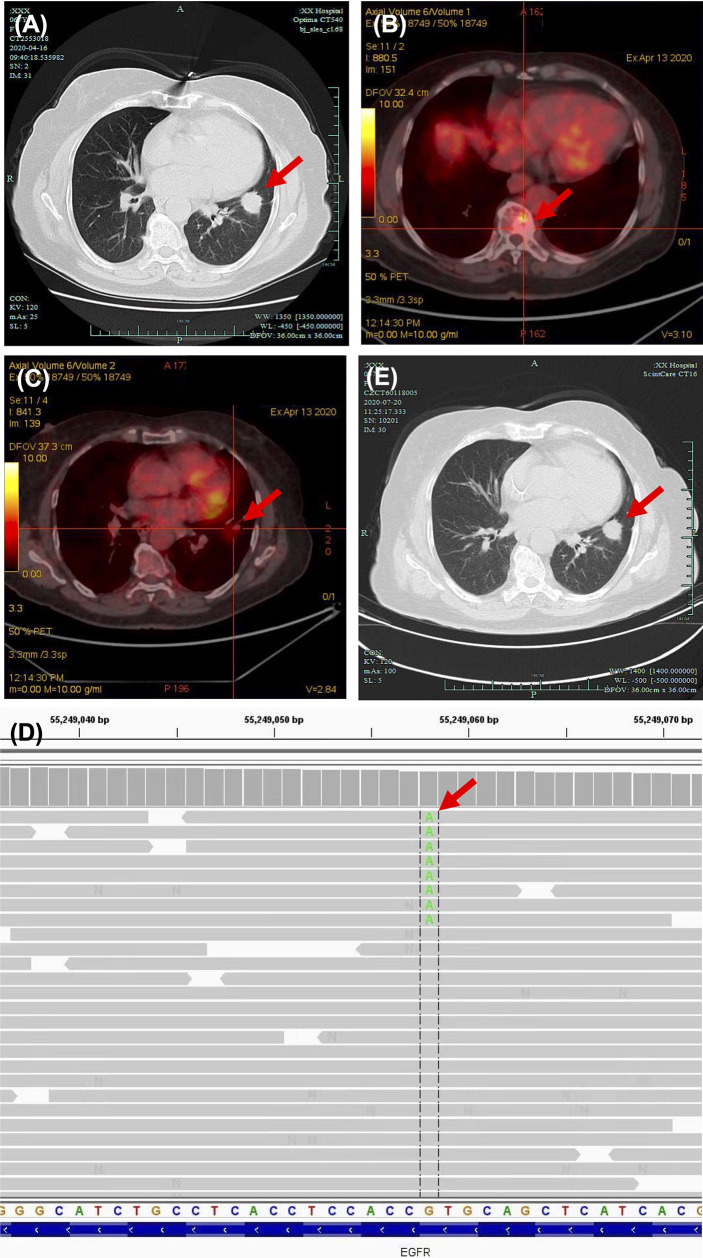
Diagnosis of EGFR V786M mutation and first-line therapy. (A) CT scan of lung window captured on April 16, 2020. Elevated SUV of bone lesion (B) and lung nodule (C) in PET-CT scan.(D) Next-generation sequencing (NGS) panel results showed an epidermal growth factor receptor (EGFR) mutation V786M in exon 20. (E) CT scan of the lung window captured on July 20, 2020, post first-line therapy. Significant abnormal findings noted (*arrow*).
